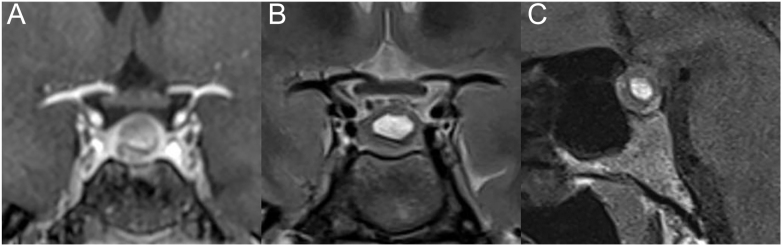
Pituitary MRI performed 10 months after diagnosis showing a stable 10 × 8 mm adenoma with necrotic hemorrhagic transformation and cystic center. (A) Post-gadolinium coronal T1-weighted image. (B) Coronal T2-weighted image. (C) Non-enhanced sagittal T1-weighted image.
